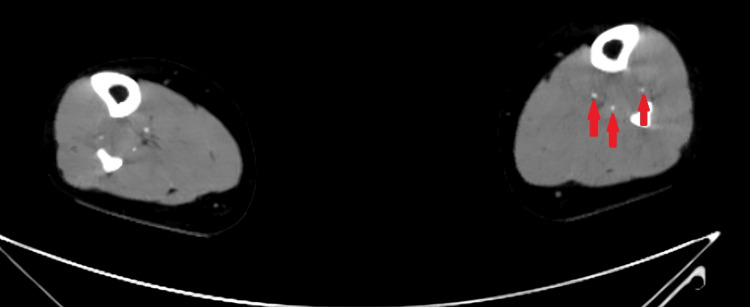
CT angiogram of distal bilateral lower extremities Arterial phase of axial post-contrast CT demonstrates that all three major arteries of the bilateral lower extremities just proximal to the ankles are patent. Arrows mark the three arteries in the left lower extremity, which is the symptomatic limb.
